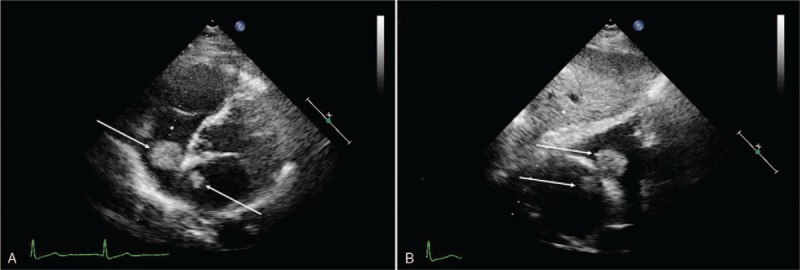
Parasternal 4-chamber view (A) and subcostal view (B) on transthoracic echocardiogram visualization of the biatrial tumor. The white arrow showing the myxoma in the right atrium and the left atrium.
